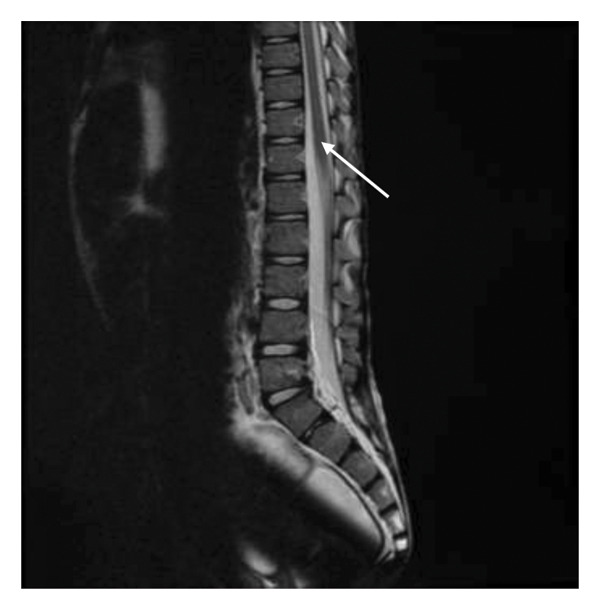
Sagittal T2‐weighted MRI shows a hyperintense fluid‐filled syrinx within the thoracic spinal cord.
